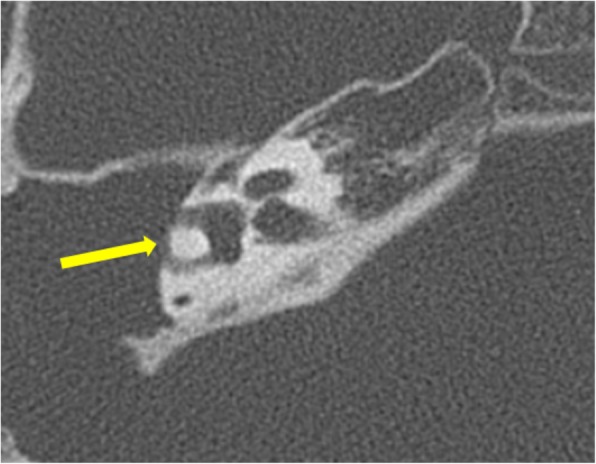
Axial CT image showing erosion of the lateral wall of the lateral semicircular canal (arrow) with the formation of labyrinthine fistula
